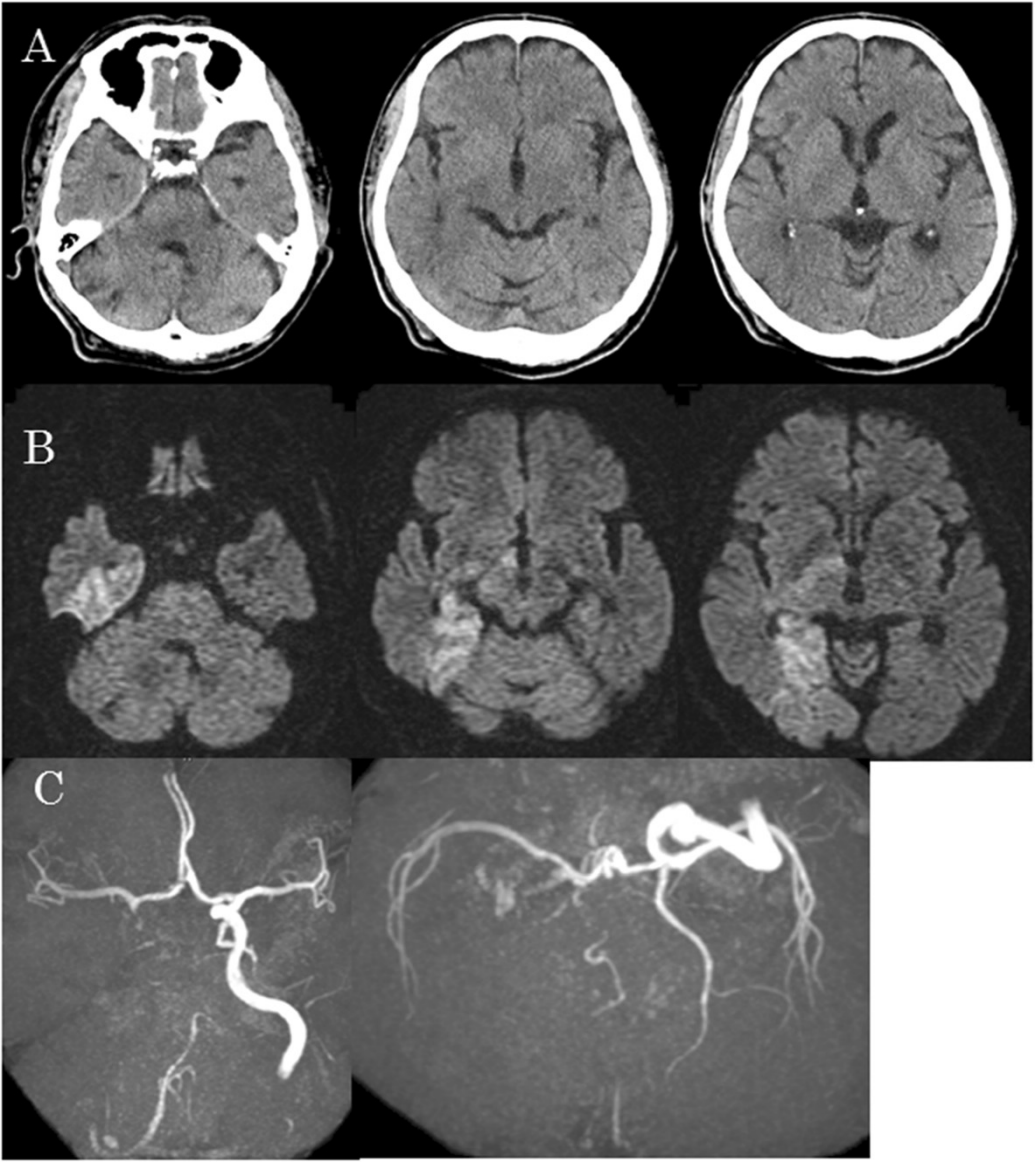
Preoperative head CT and head MRI/MRA. a Initial head CT showed no early ischemic signs. b The onset head MRI DWI showed HIA in the right medial temporal lobe, right hypothalamus, right internal capsule and right medial occipital lobe. c Initial head MRA showed right ICA occlusion and BA occlusion. However, right anterior and middle cerebral artery were represented clearly by virtue of the Acom
